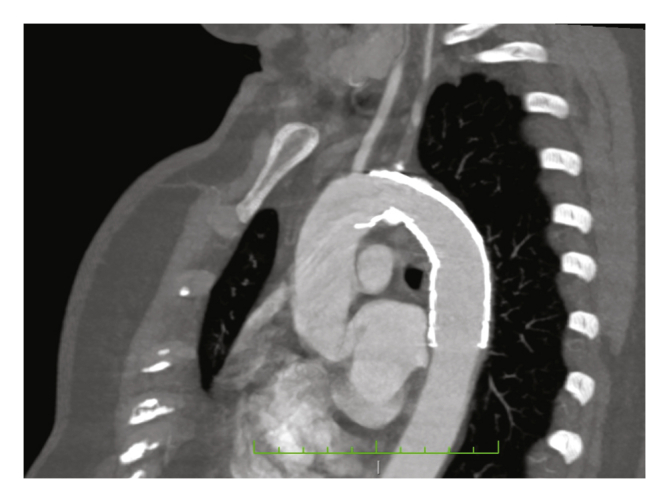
Follow up CT scan (2 months).
